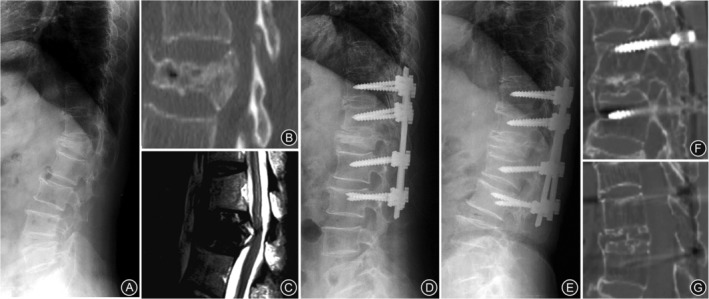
(A) Preoperative radiograph of a 71‐year‐old woman with L_1_ osteoporotic compression fracture accompanying functional paraplegia showing a kyphotic angle of 23.5 degrees. (B) A sagittal CT scan showing collapse of the vertebral body. (C) A sagittal T2WI magnetic resonance image showing cord compression. (D) Immediate postoperative radiograph showing that the kyphotic angle decreased to 10.4 degrees. (E) A radiograph taken at 5 years postoperative shows increased kyphosis to 27.3 degrees (16.9‐degree correction loss). However, the patient's pain relief and partial recovery of functional paraplegia were maintained. (F, G) CT scan taken at 5 years postoperative showing bone union of the osteotomy site and decompression of the spinal canal (F, intervertebral foramen area; G, central area).
